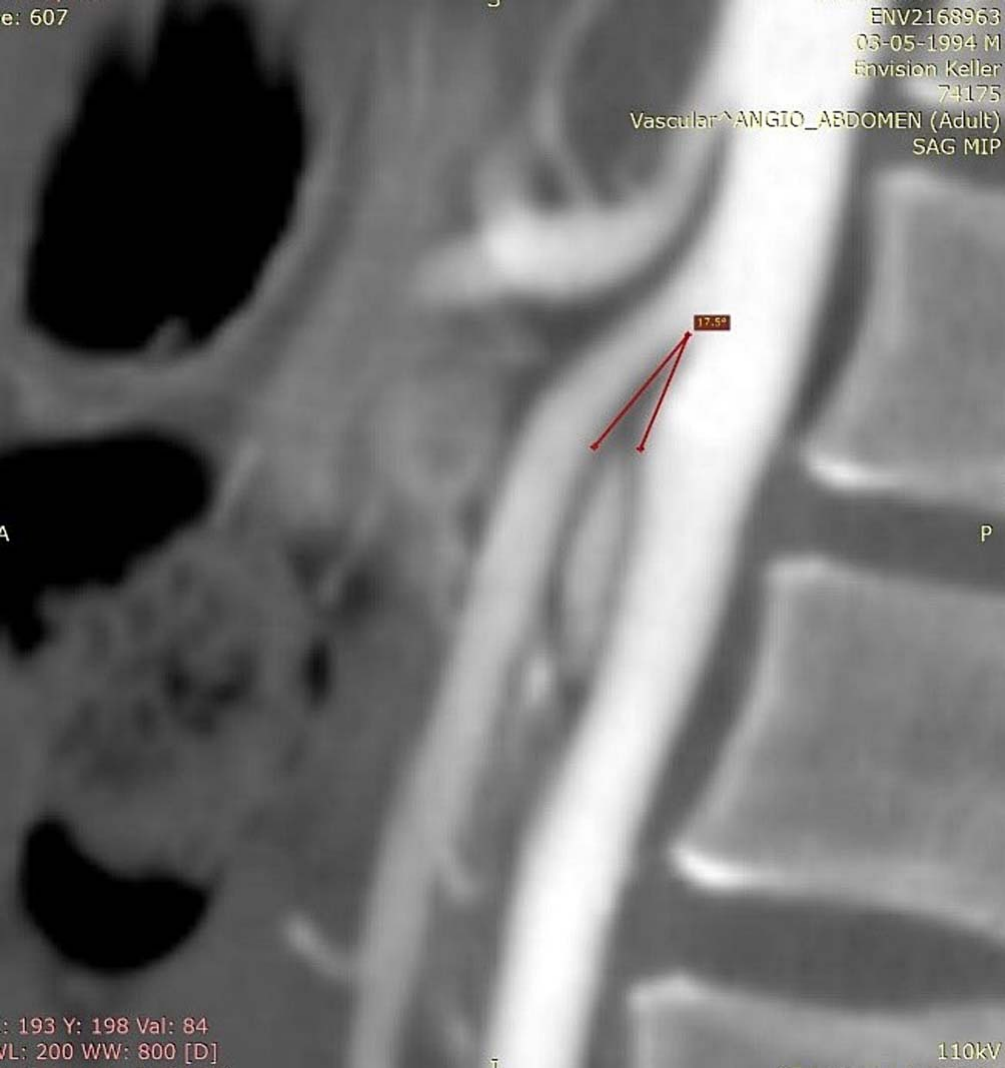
Computed tomography. Aortomesenteric angle of 17.5°.
